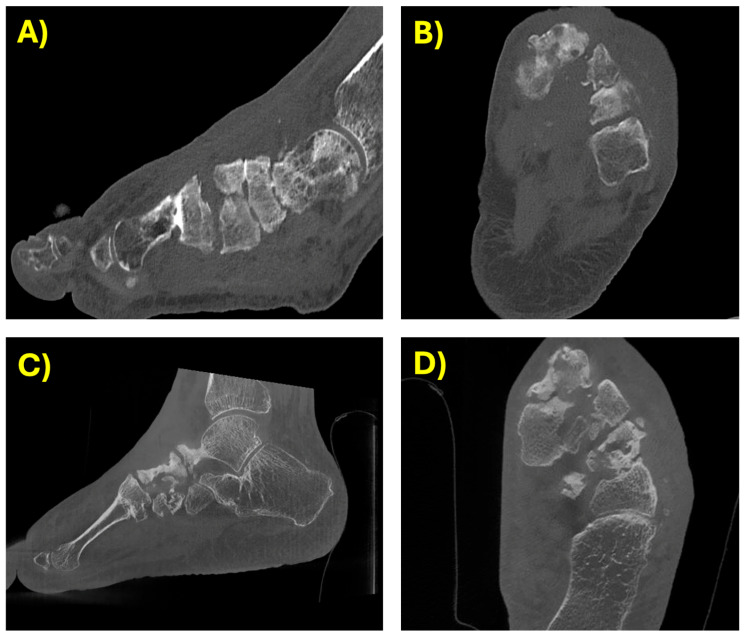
Timeline of the CT scan evolution. Panels (A,B) 19 August 2021: alteration of the dorsal bone and irregular thickening of the spongy bone of the first cuneiform, with irregular contours at the level of the joint between the first metatarsal and the first cuneiform. This finding may be compatible with osteomyelitis originating from a trophic lesion of at least 2 cm on the dorsal aspect of the midfoot. Panels (C,D) 13 January 2023: well-healed fracture of the first metatarsal, although the cortical bone on the inferior aspect, at the middle-proximal third, remains interrupted, and there is marked sclerosis of the fused fragments. The spongy bone of the midfoot remains significantly sclerotic, consistent with the sequelae of a past infection. No new erosive changes are evident.
